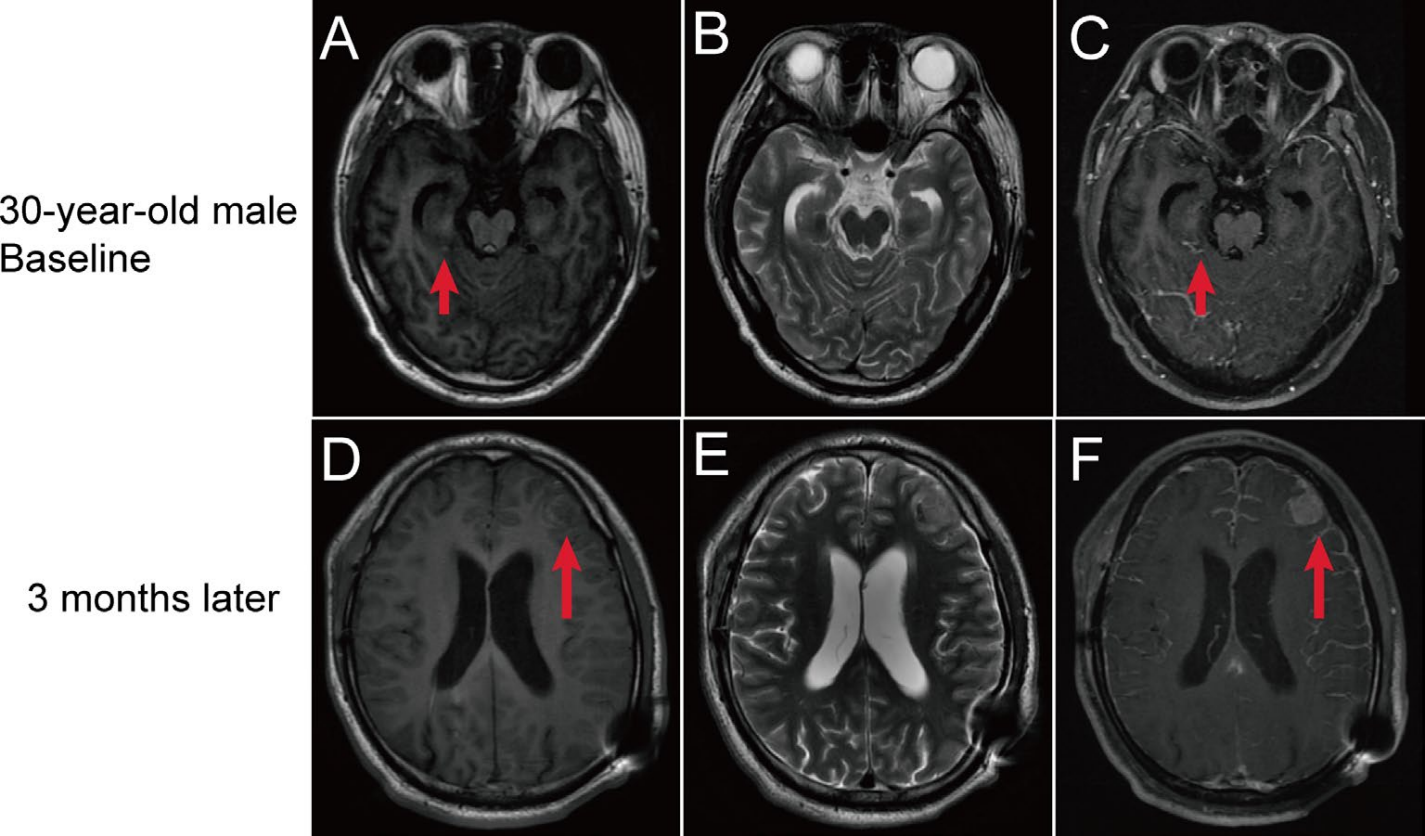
(A–C) Brain MRI at 30 years of age, after the onset of neurological symptoms, shows a patchy region with slightly hypointense T1 signals (A) and slightly hyperintense T2 signals (B) in the uncus of the right parahippocampal gyrus; leptomeningeal enhancement is markedly increased on T1 FLAIR after contrast enhancement (C). (D–F) Follow‐up MRI performed 3 months later reveals a nodular lesion in the left frontal lobe with isointense to slightly hypointense T1 signals (D) and isointense to slightly hyperintense T2 signals (E); the lesion has a well‐defined border and demonstrates prominent, homogeneous enhancement on T1 FLAIR contrast‐enhanced imaging (F).
